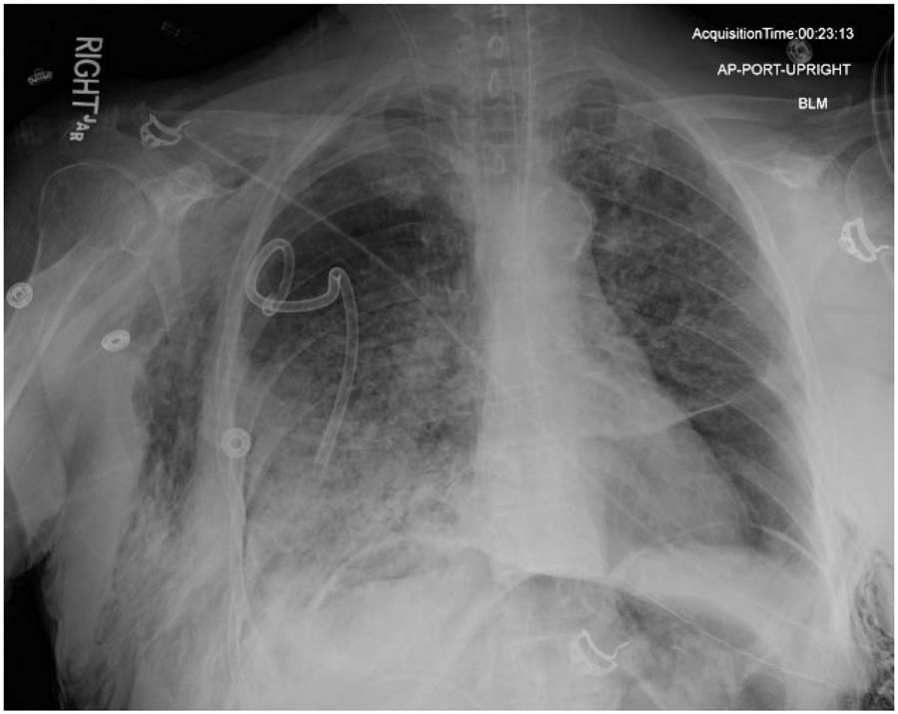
AP upright chest radiograph showing intraabdominal free air, right-sided chest tube in place, and bilateral chest wall subcutaneous emphysema that is worse on the right. Bilateral patchy airspace disease is noted as well.
